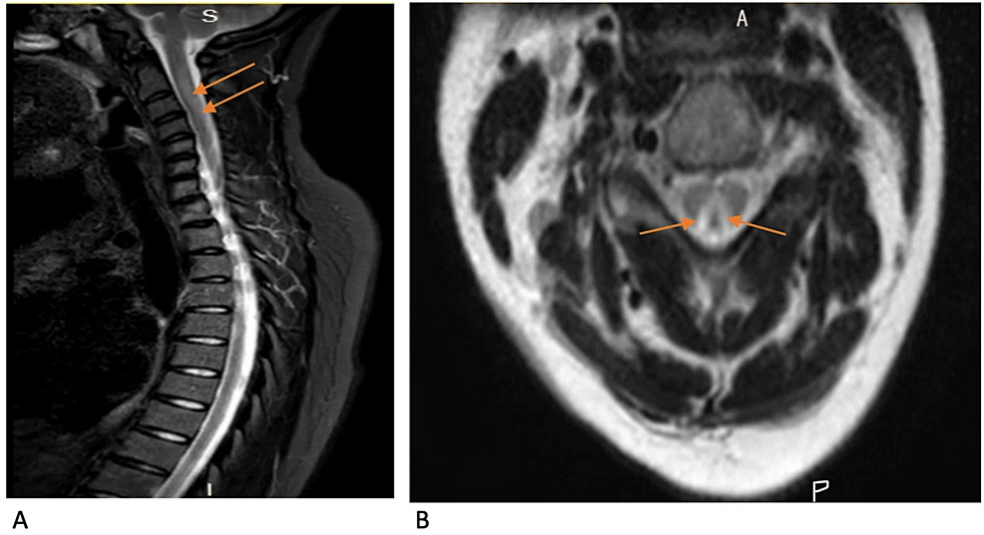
MRI spine of the index patient. (A) Sagittal section showing T2 hyperintensity in the dorsal cervical spinal cord indicated by orange arrows. (B) Axial section showing T2 hyperintensity with inverted V sign in cervical spinal cord indicated by orange arrows.
